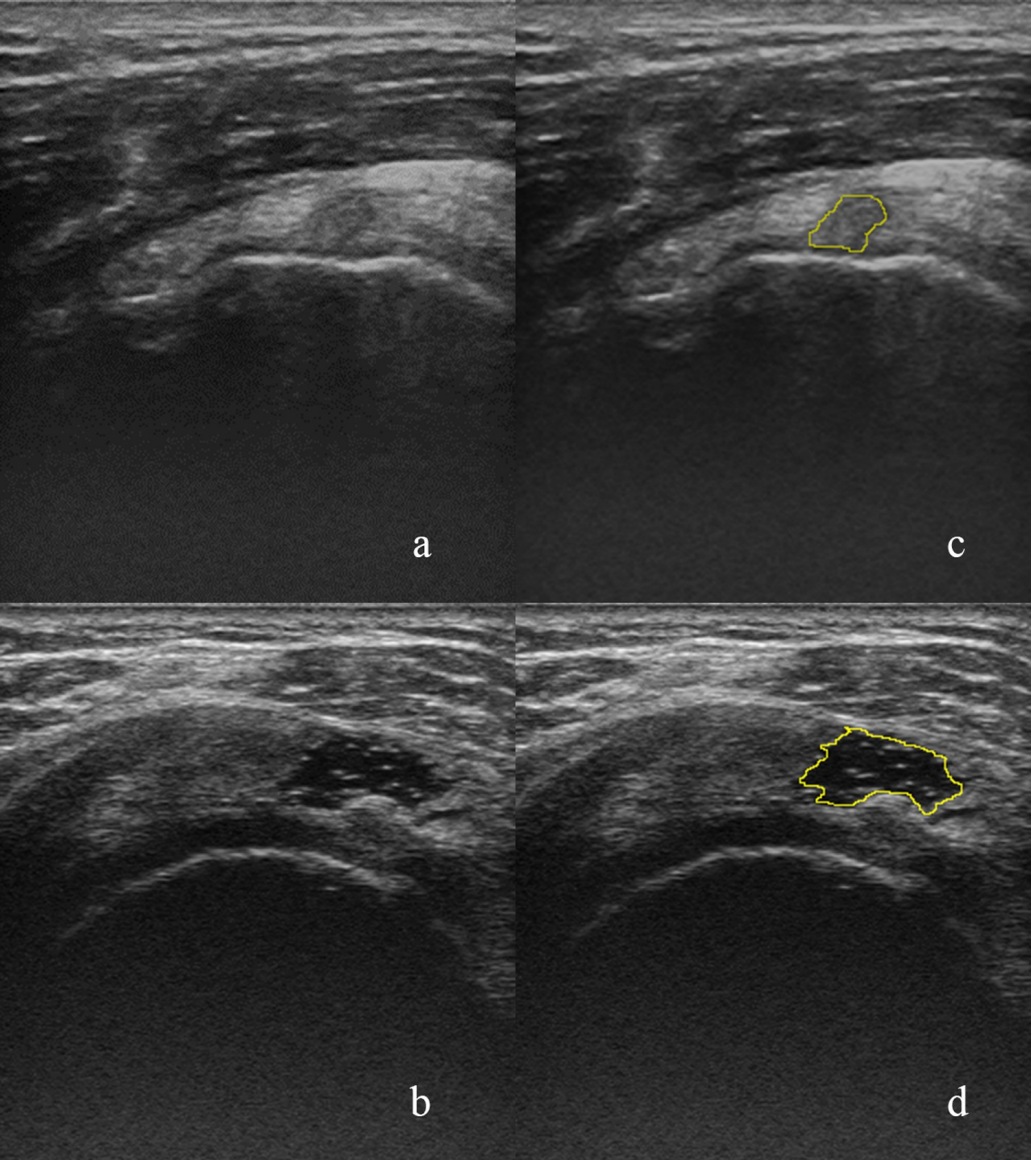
Supraspinatus tendon shown in ultrasound images. (a) A case of tendon tendinopathy. (b) A case of supraspinatus tear. (c) and (d): The lesion contours of (a) and (b), respectively, which were delineated by a shoulder orthopedic surgeon using ImageJ.
